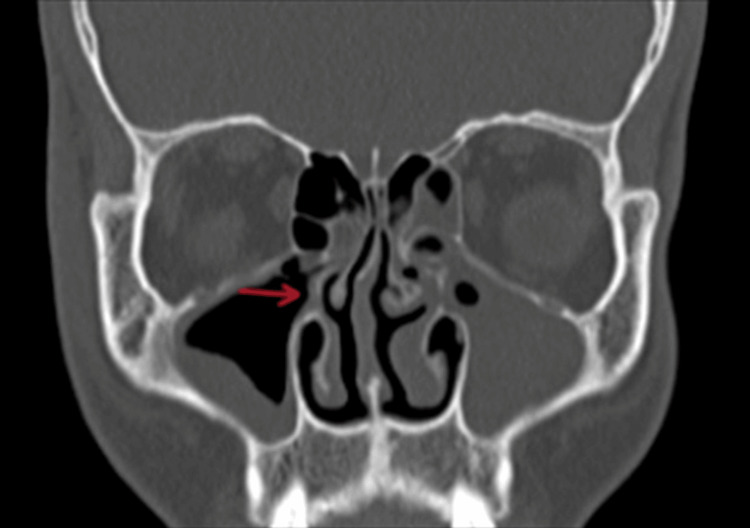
CT showing attachment of the right uncinate process to the middle turbinate (indicated by a red arrow).
